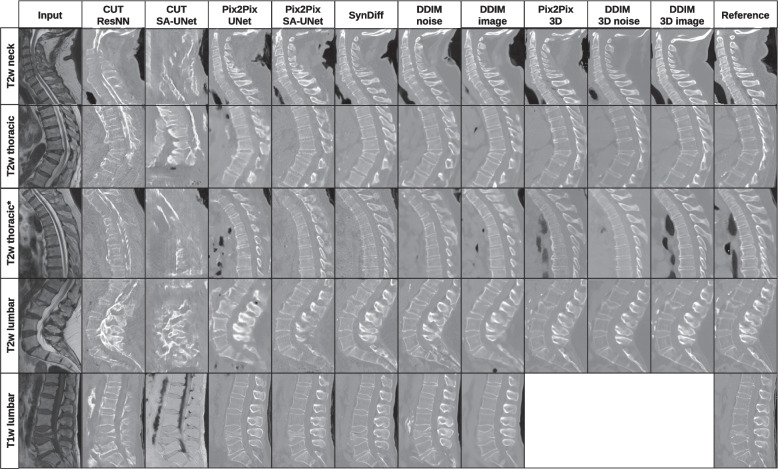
Translation from test sets T1-weighted/T2-weighted MRI to CT from the neck to the lumbar vertebra. We did not control the type of reconstruction of the CT. Therefore, the noise level and appearance could differ from the reference and were still considered correct. The 3D variances were trained on an improved training set, which was only done for T2 weighted. The reference is a registered real CT. * is an off-angle acquisition with strong partial volume effects. The dataset contains a high number of broken vertebral bodies, which causes them to be also translated correctly. *CUT* Contrastive unpaired translation, *DDIM* Denoising diffusion implicit model, *SA-UNet* Self-attention U-network
